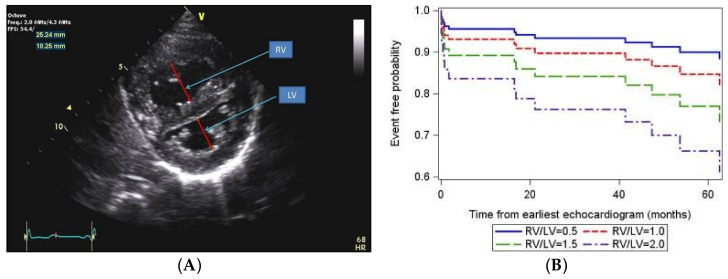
(A) Parasternal short axis view of the right and left ventricles (RV/LV) at the level of the papillary muscles. The RV/LV ratio is derived from RV diameter and LV diameter at end-systole. RV/LV end-systolic ratio is predictive of outcome; (B) Estimated survival curves for four possible RV/LV ratios estimated from the Cox varying coefficients regression corresponding to a hazard ratio of 2.49 for RV/LV ratio [66].
